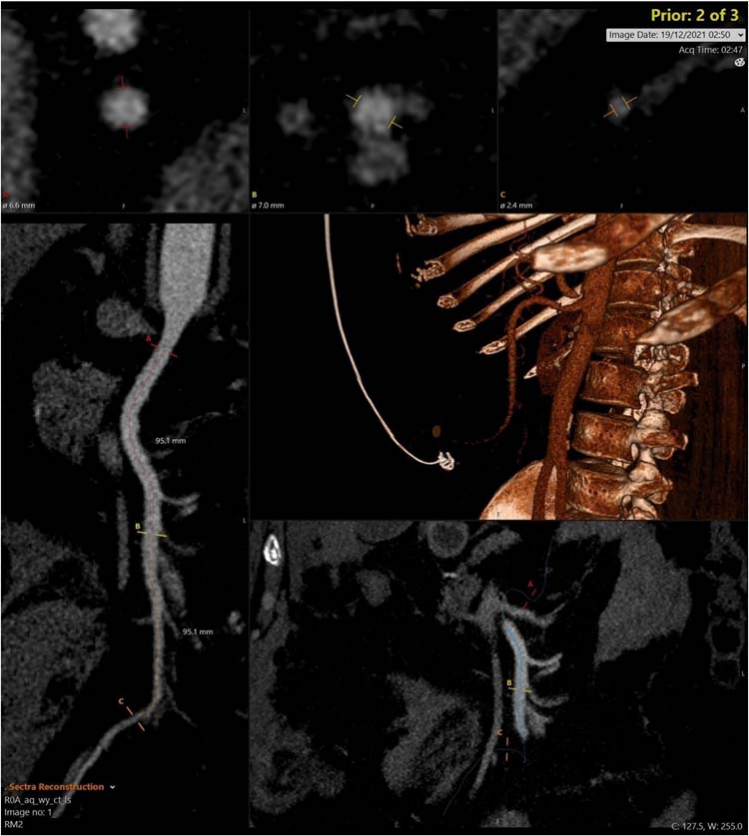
Curved reprogramming of the initial CT angiogram. Curved reprogramming of the patient’s initial CT angiogram showed no evidence of an aneurysm within the superior mesenteric artery, effectively ruling this out as a cause of the patient’s acute bleed.
